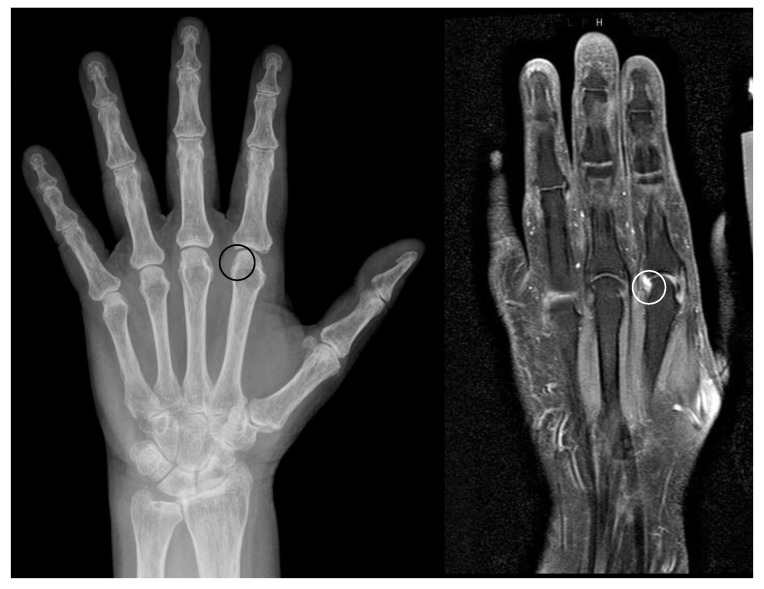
Erosions within pseudoerosions. Example of the development of an erosion within a pseudoerosion (black/white circle) in a left hand of a 65 years old female patient with longstanding mild seropositive rheumatoid arthritis. Left: radiograph, right: MRI.
